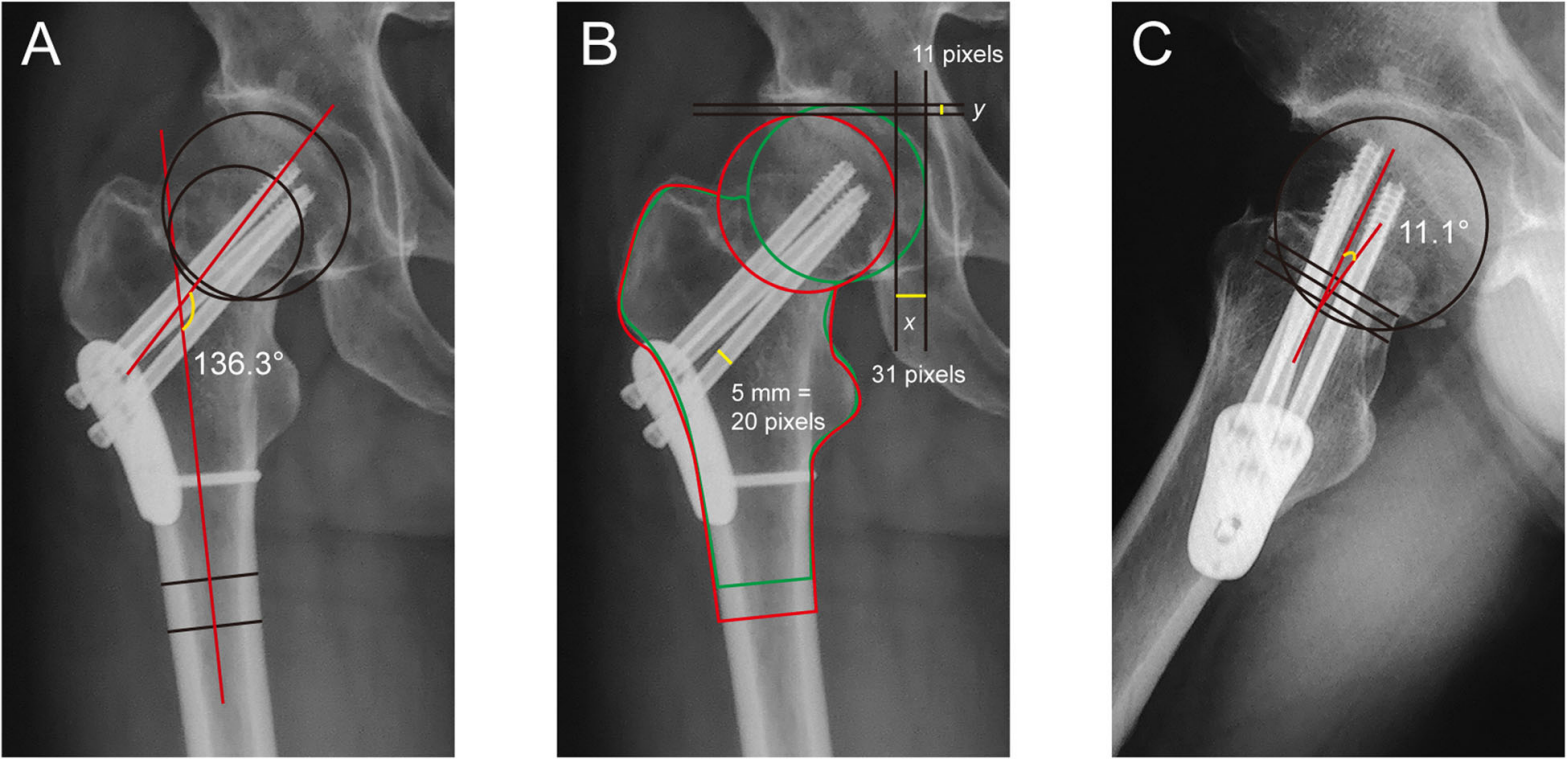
Proximal femoral plating of a 53-year-old male with retroverted femoral neck fracture (Garden type III). a Preoperative X-ray. b Three-dimensional reconstruction of CT scans. c, d Intraoperative anteroposterior and lateral radiographs of definitive fixation (posterior tilt: 4.7°). Postoperative radiographs at 1 month (posterior tilt: 11.1°) (e, f), 6 months (g, h), and 12 months (i, j)
